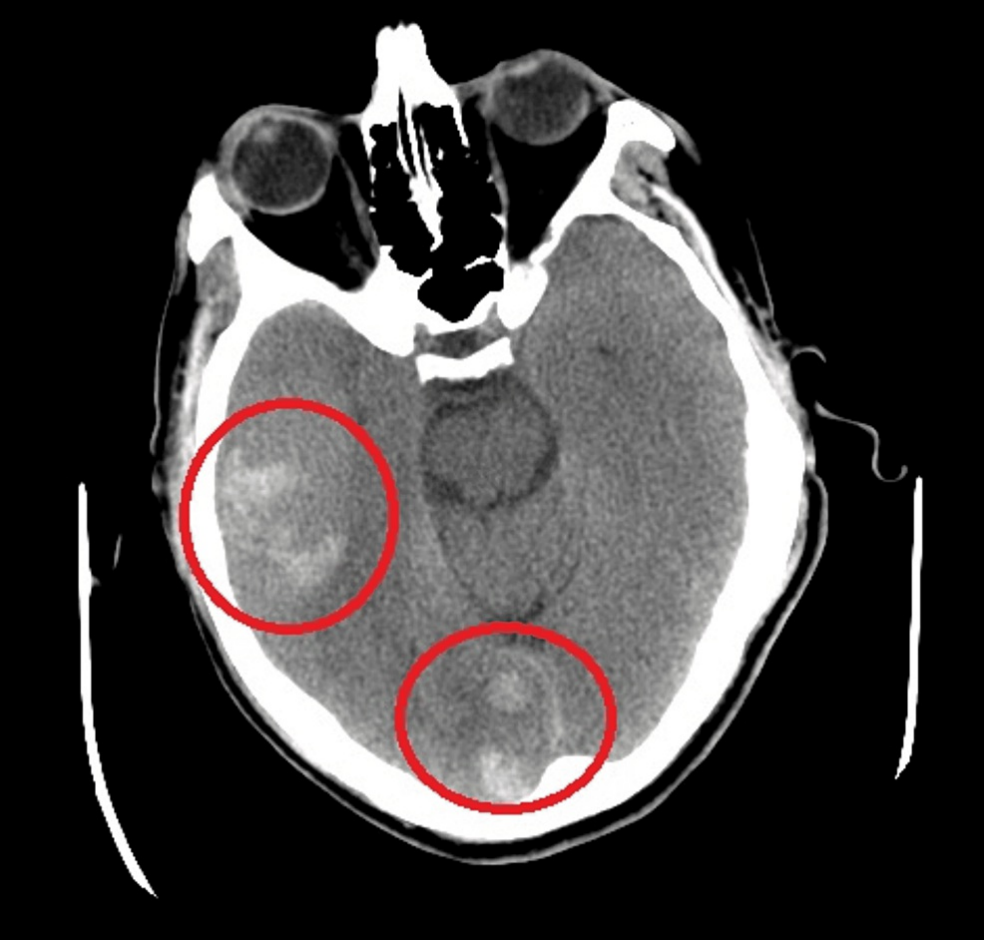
CT of the brain showing multifocal, right hemispheric, and right cerebellar haemorrhages with associated signs of a subarachnoid haemorrhage. Red circles show areas of haemorrhage.
